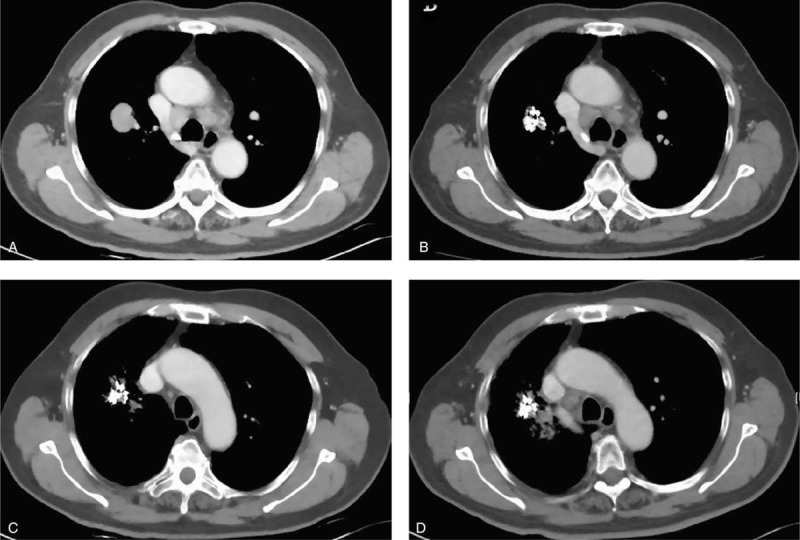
A 70-year-old male patient. (A) CT scan before treatment, (B–D) 1, 2, and 3 months after ^125^I implantation treatment, the lesion apparently shrunk (CR). The particles gathered and did not shift to the normal lung. CT = computed tomography, CR = complete response.
